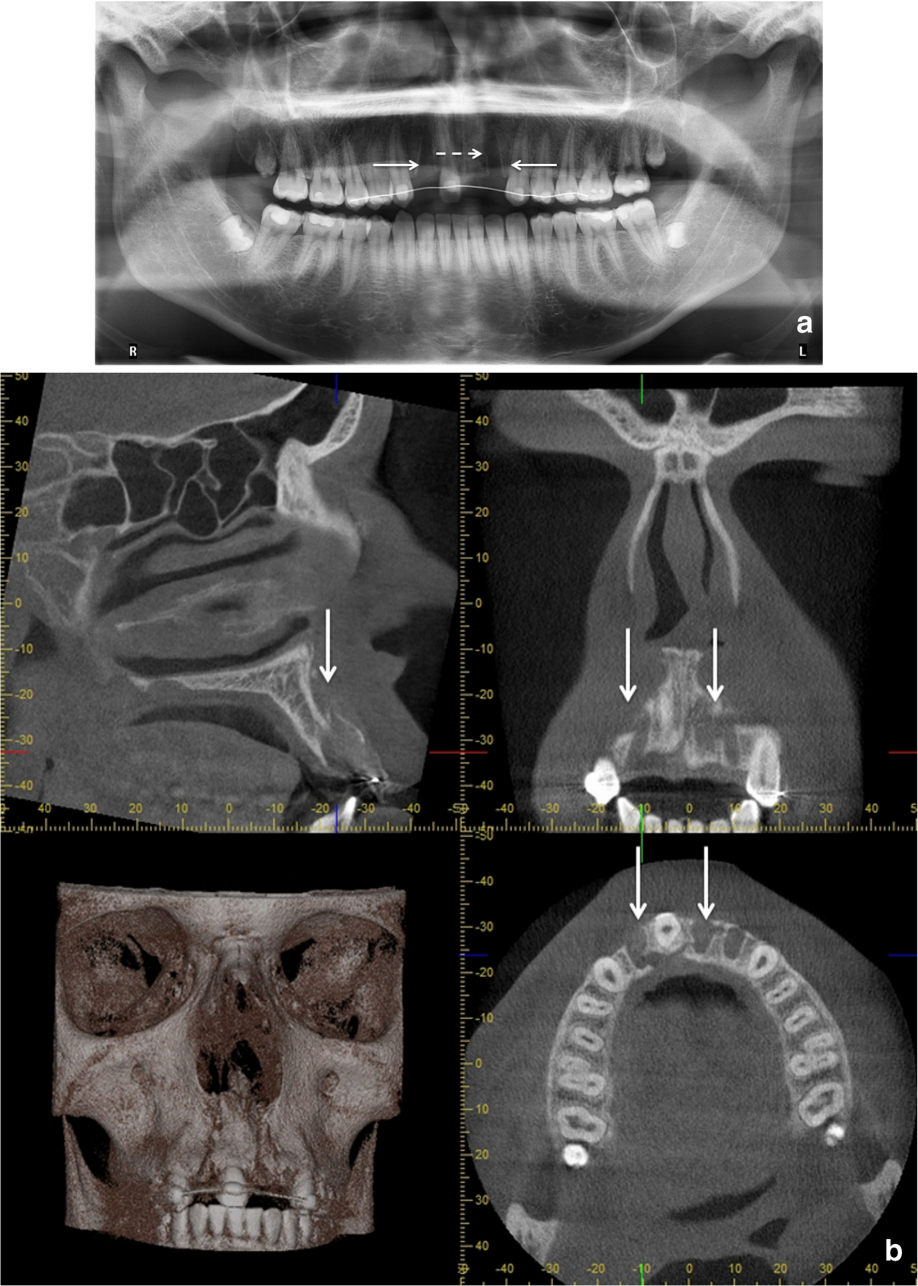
The patient had been in a motorcycle accident. (a) Dd. 12, 21 and 22 have been exarticulated and their root sockets are visible in the PTG (*horizontal arrows*). Alveolar fracture (*dashed arrow*) is not so easily visible in PTG in comparison to the CBCT examination where the alveolar fracture with dislocation is evident (*vertical arrows*) (b). (Courtesy Tapio Tammisalo.)
